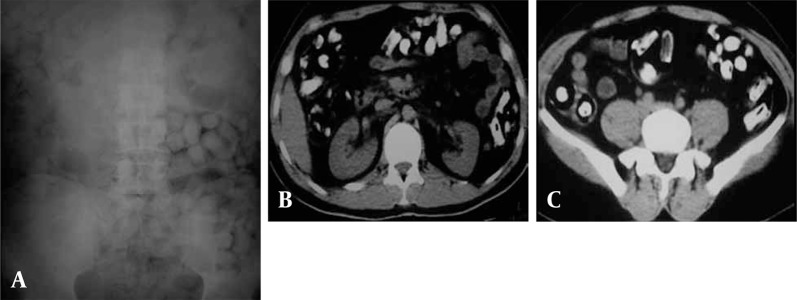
A, Abdominopelvic plain x-ray reveals several uniform radiopaque packets; B-C, Abdominal CT scan without oral contrast shows numerous randomly distributed packets within the small bowel and colon. Their density is 150-170HU which is compatible with the density of opium.
